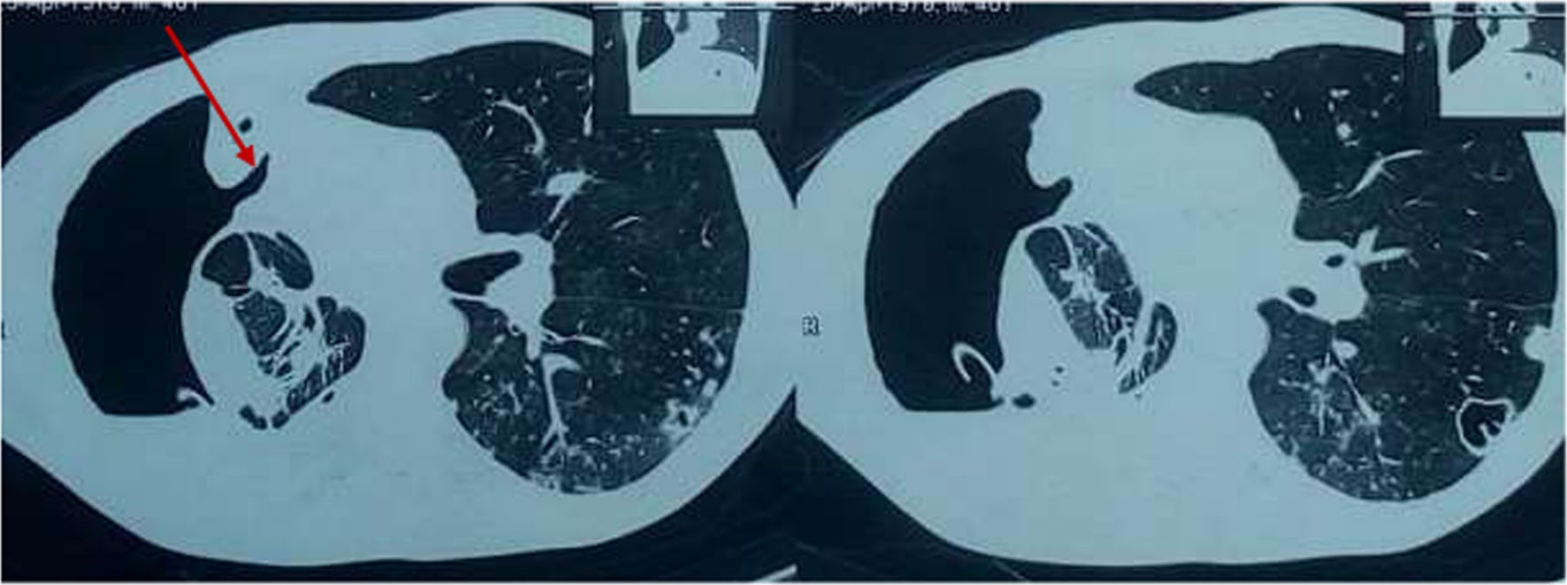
Computed tomography scan segments showing bronchopleural details. Computed tomography scan segments showing details of bronchopleural fistula in the right hemithorax (arrow), pyo-pneumothorax, partial right middle and lower lung lobe collapse, and thick-walled cavity in the right upper lobe of the right hemithorax
